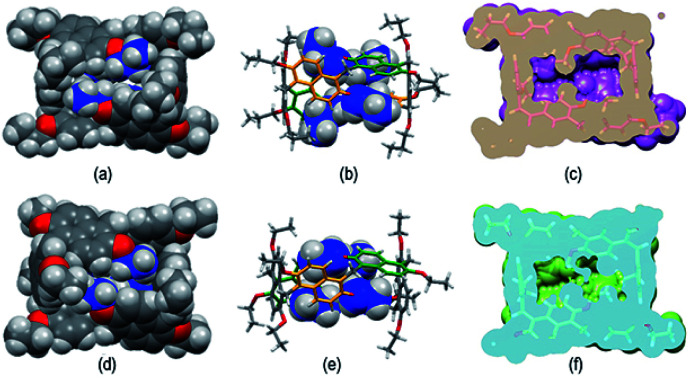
Self-filling of the cavities of prism[6]arenes as obtained by X-ray structures. (a) and (b) Different views of PrS[6]nPr. (d) and (e) Different views of PrS[6]Et. (c) and (f) Self-filling of the cuboid cavity. Cross sections of the PrS[6]Et (f) and PrS[6]nPr (c) molecules. An enclosed internal cavity was obtained from accessible surface area calculations using a 0.4 Å probe. The free cavity volume is 49 Å^3^ and 77 Å^3^ for PrS[6]Et and PrS[6]nPr, respectively.
